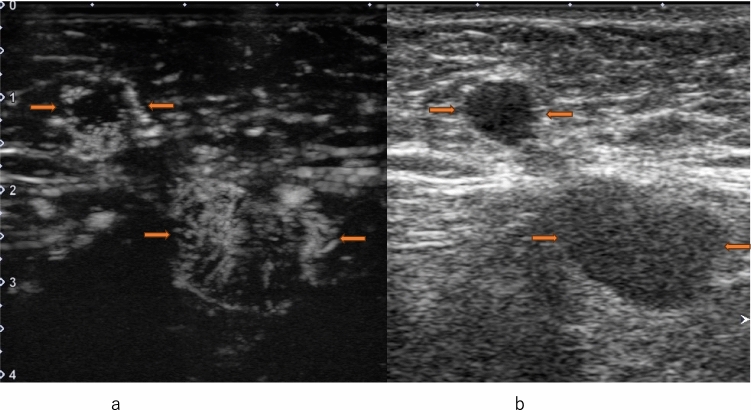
Metastatic lymph node. a Contrast-enhanced ultrasound shows heterogeneous enhancement with a clear defect (between arrows). b Conventional ultrasound shows an axillary lymph node with cortical thickening (between arrows)
